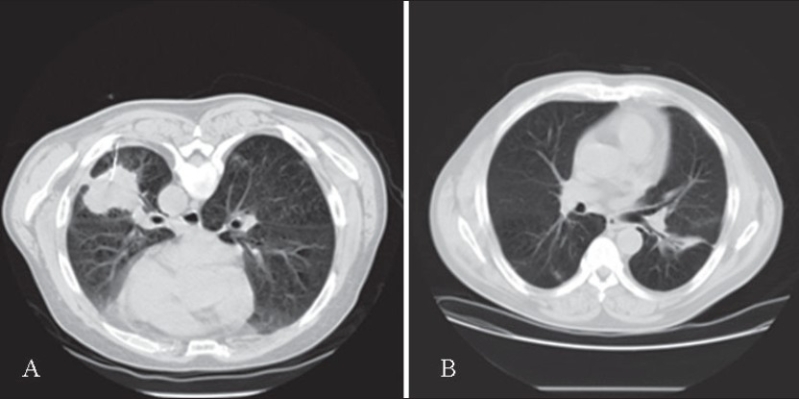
(A) Prone CT chest, placement of 22G Westcott biopsy needle in the left lower lobe mass. (B) Follow up CT chest two months after biopsy. Notice marked improvement with residual inflammation and focal bronchiectasis in the left lower lobe.
